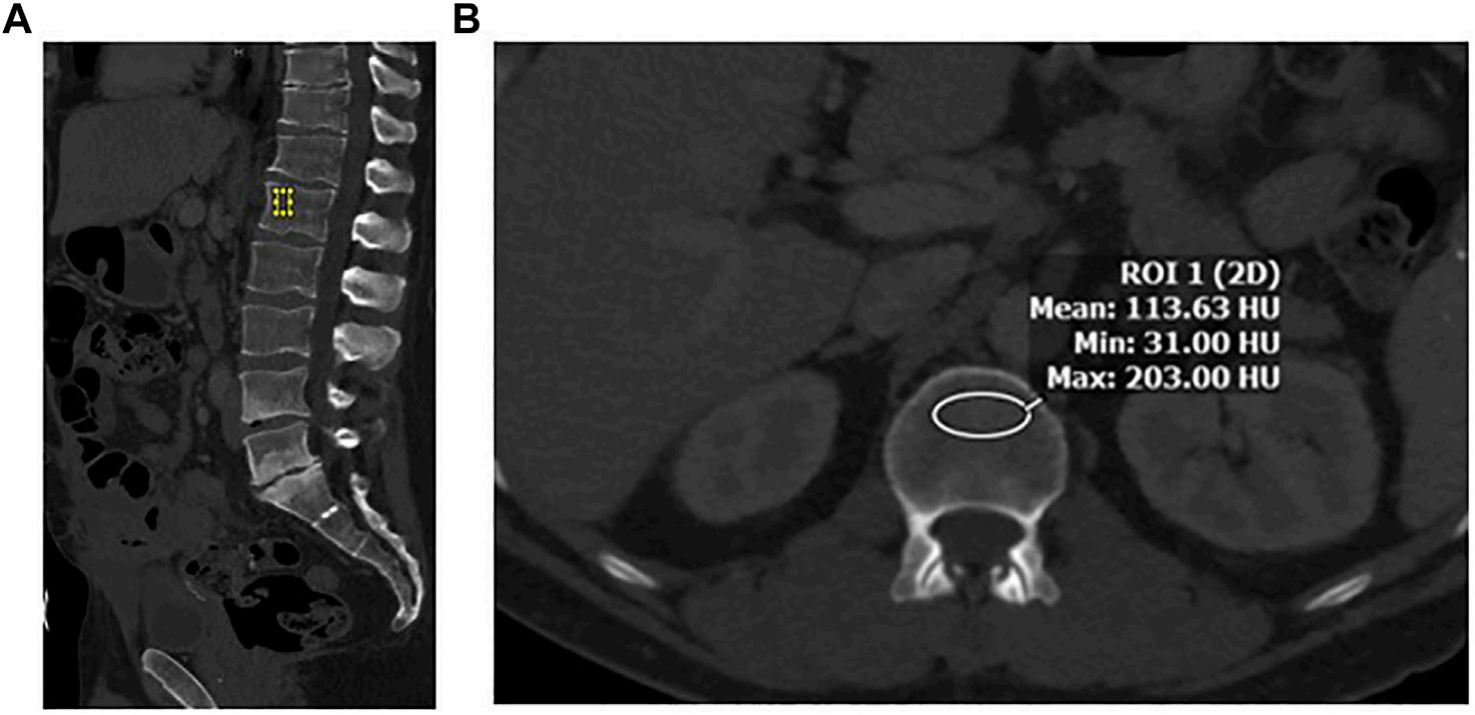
(A) Sagittal image in bone windows demonstrating placement of an elliptical region of interest at L1 along the anterior aspect of the vertebral body. (B) Axial image at the L1 level along the superior aspect, with an elliptical region of interest to measure CT-derived bone density in Hounsfield Units (HU).
